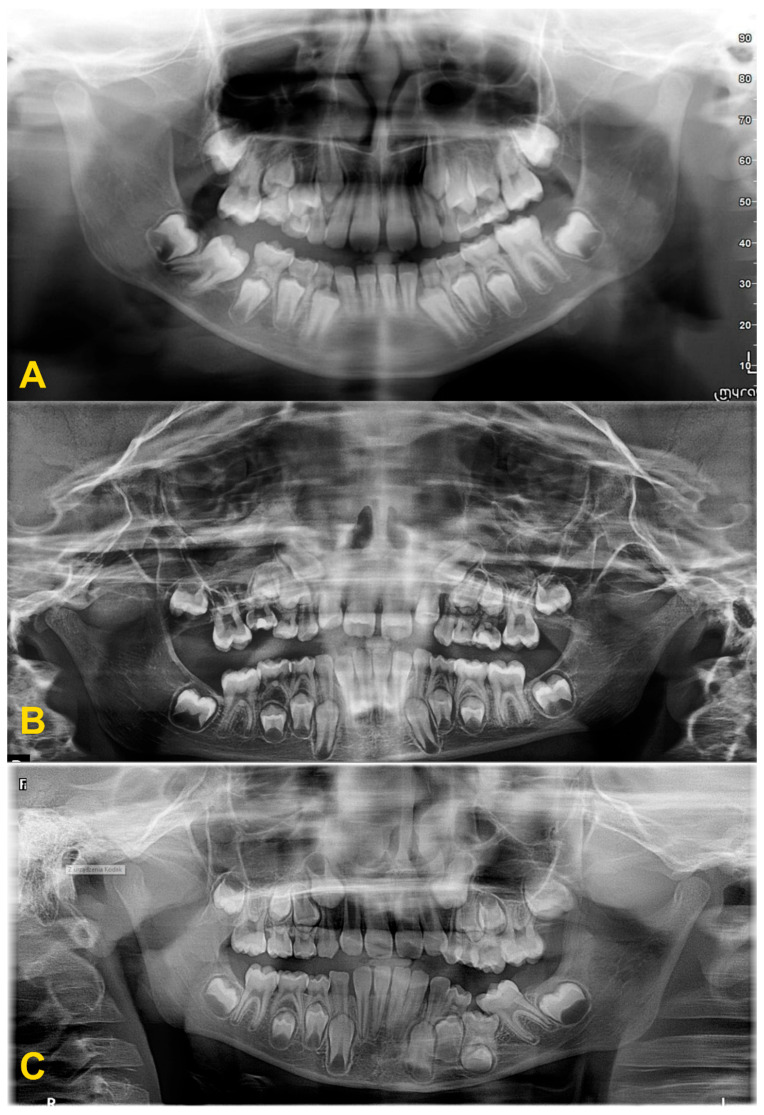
Panoramic X-ray examinations: (A) mild infraocclusion of tooth no. 55 (immersion of 1–2 mm); (B) moderate infraocclusion of tooth no. 55 (infraposition of 2–3 mm), displacement and growth delay of permanent successor, and angulation of the adjacent teeth with loss of space; and (C) severe infraocclusion of tooth no. 75 (immersion of more than 3 mm), displacement and growth delay of permanent successor, severe angulation of the adjacent teeth with loss of space (the same patient as in Figure 6C).
